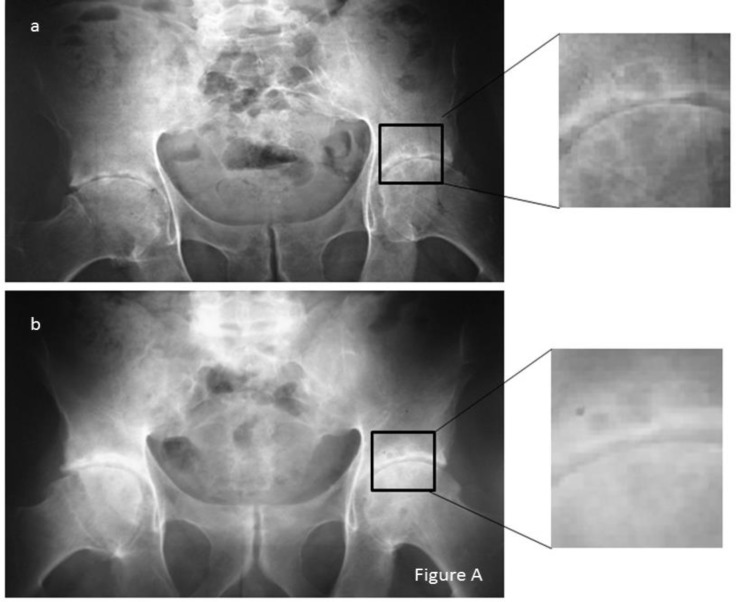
Radiographic images of anteroposterior x-rays of the pelvis in 2010 (a) and in 2014 (b) of case 1 showing reduction of the space narrowing with less subchondral cysts
